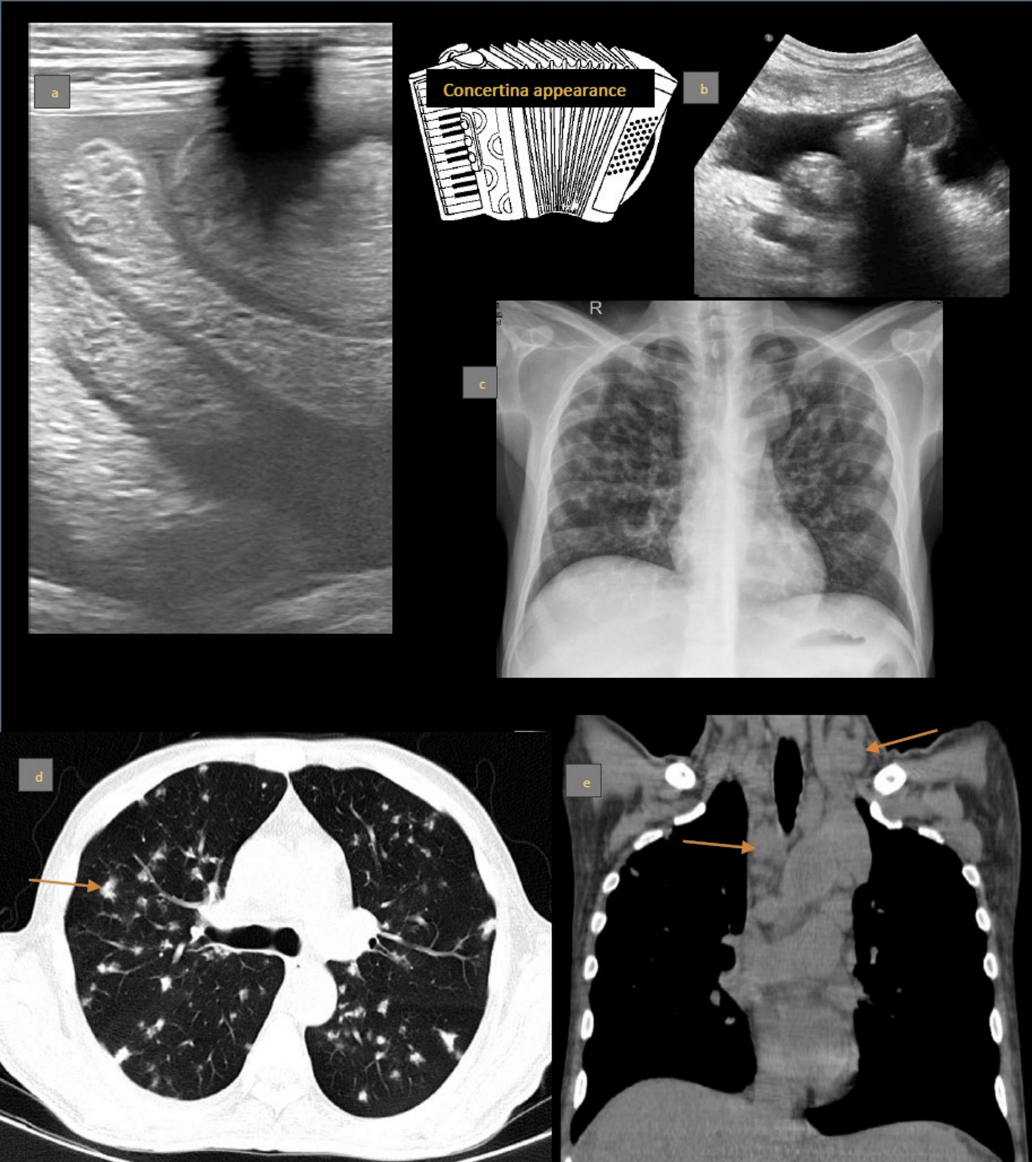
a: Ultrasound showing stacked small bowel loops with a concertina-like appearance. b: Ultrasound showing central clustering of small bowel loops at the mesentery root, resembling a cauliflower. c: Chest radiograph showing patchy nodular opacities in both lungs. d: Axial HRCT showing peribronchovascular nodules. e: Coronal CT of the chest with enlarged mediastinal and neck nodes. HRCT: high-resolution computed tomography, CT: computed tomography
